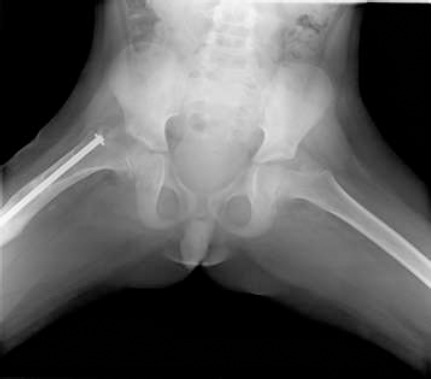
anteroposterior hip X-ray—right hip is in place at cast removal
